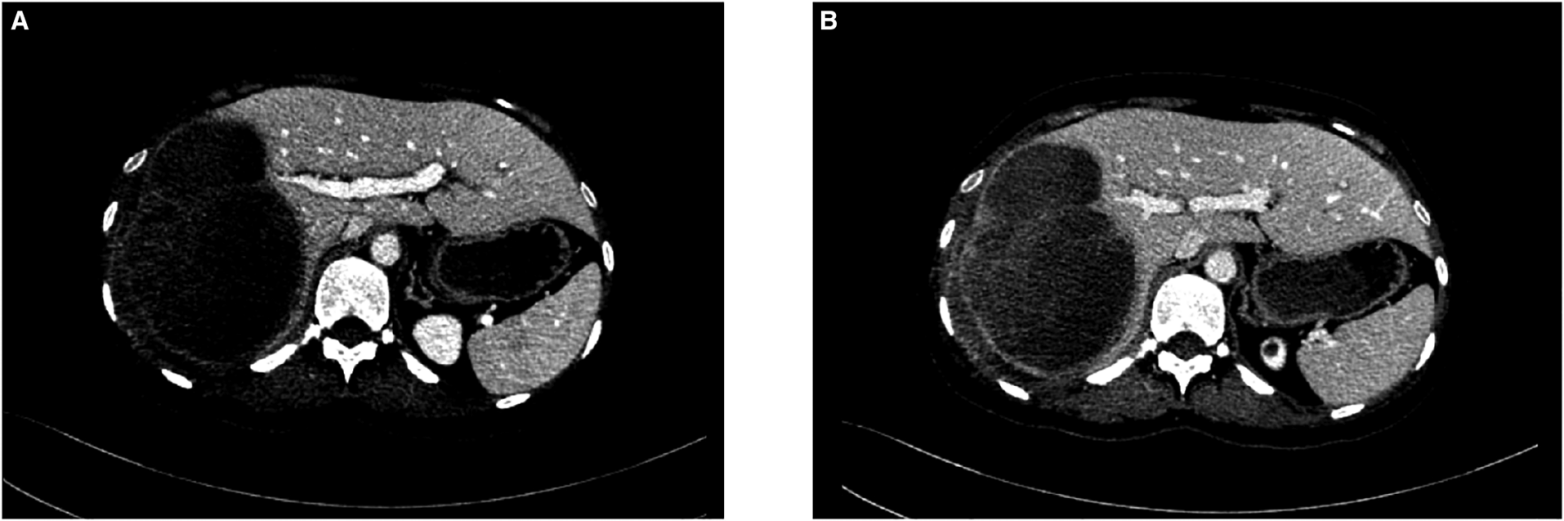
Preoperative abdominal CT findings (A) arterial phase (B) venous phase.
